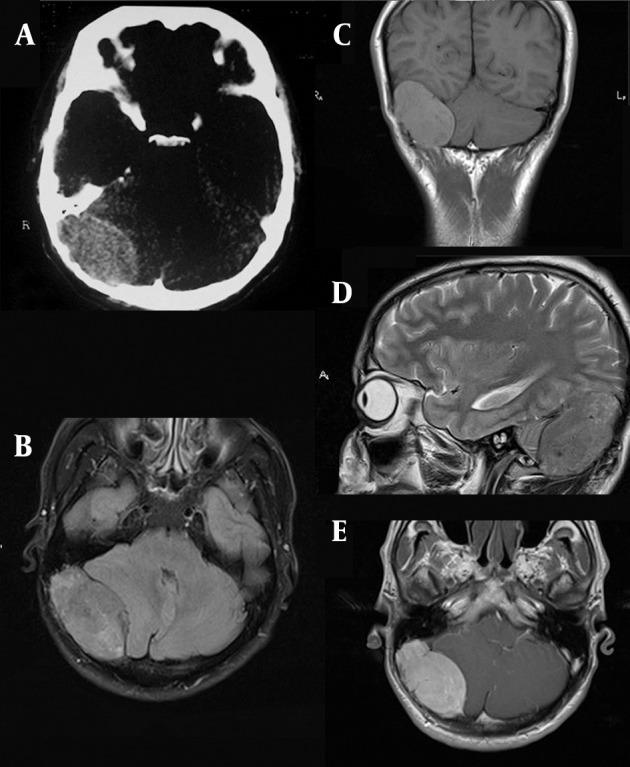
A 37-year-old man with headache, large extraaxial iso-signal space occupying lesion on the right side of the posterior fossa with erosion of the internal table and compressive effect to the right cerebellar hemisphere and marked enhancement. A, CT image with erosion of the internal table; B, Axial FLAIR MRI with isosignal extra axial SOL at the right side of the posterior fossa; C, Coronal T1 WI without contrast; D, Sagittal T2 WI; E. Axial post-contrast T1 WI.
